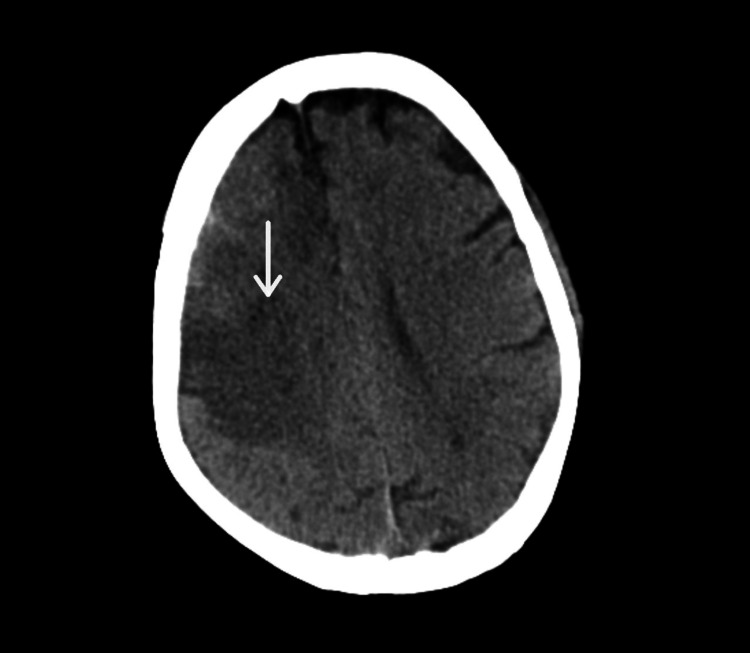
Computed tomography (CT) of the brain Large infarct in right Fronto-Parieto-Temporal lobes (white arrow)
